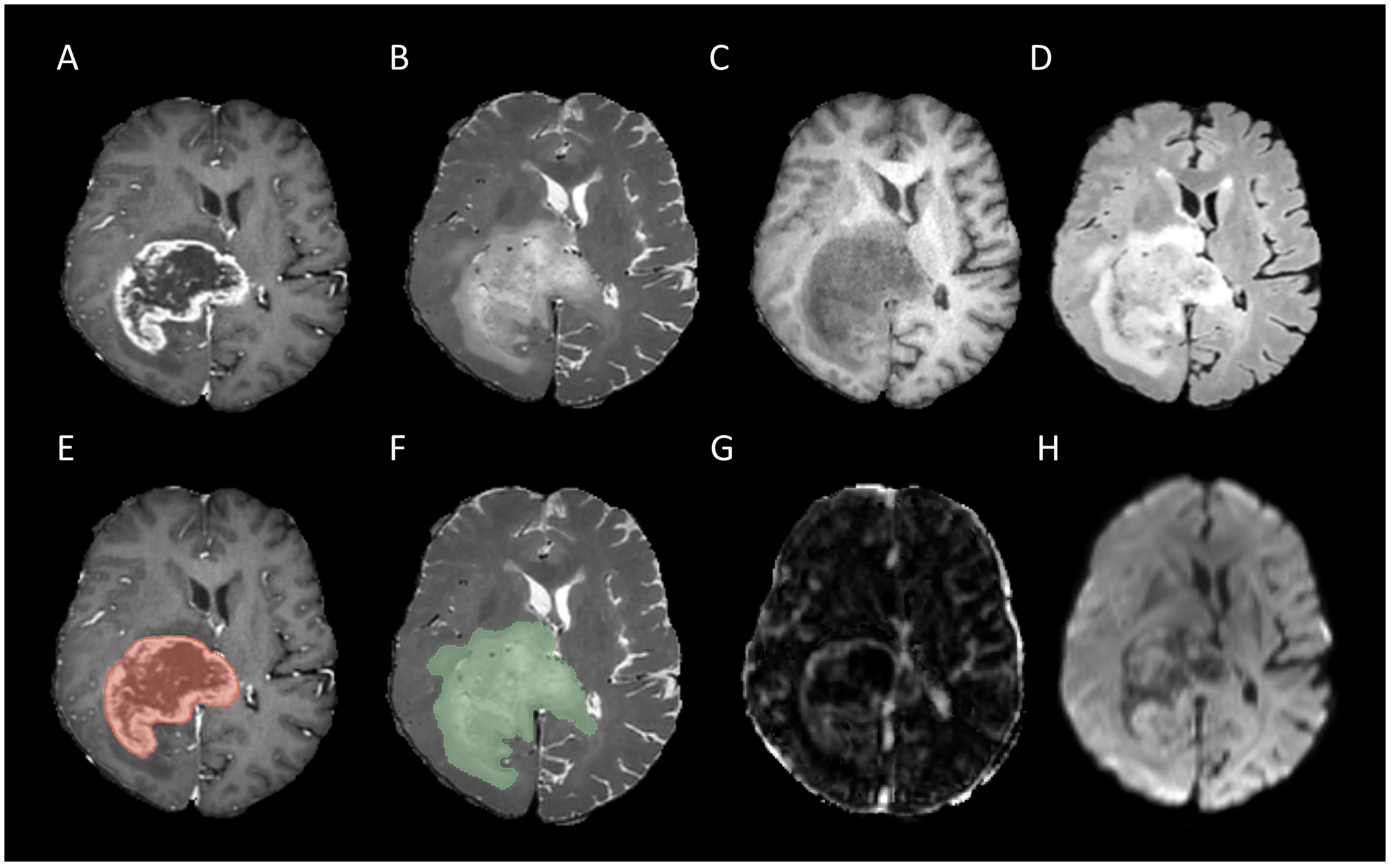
Example for MR images of a patient with MGMT unmethylated: T1CE (A), T2WI (B), T1WI (C), FLAIR (D), tumor core segmentation on T1CE (E), whole tumor segmentation on T2WI (F), Ktrans map of DCE (G), and MD map of DTI (H).
